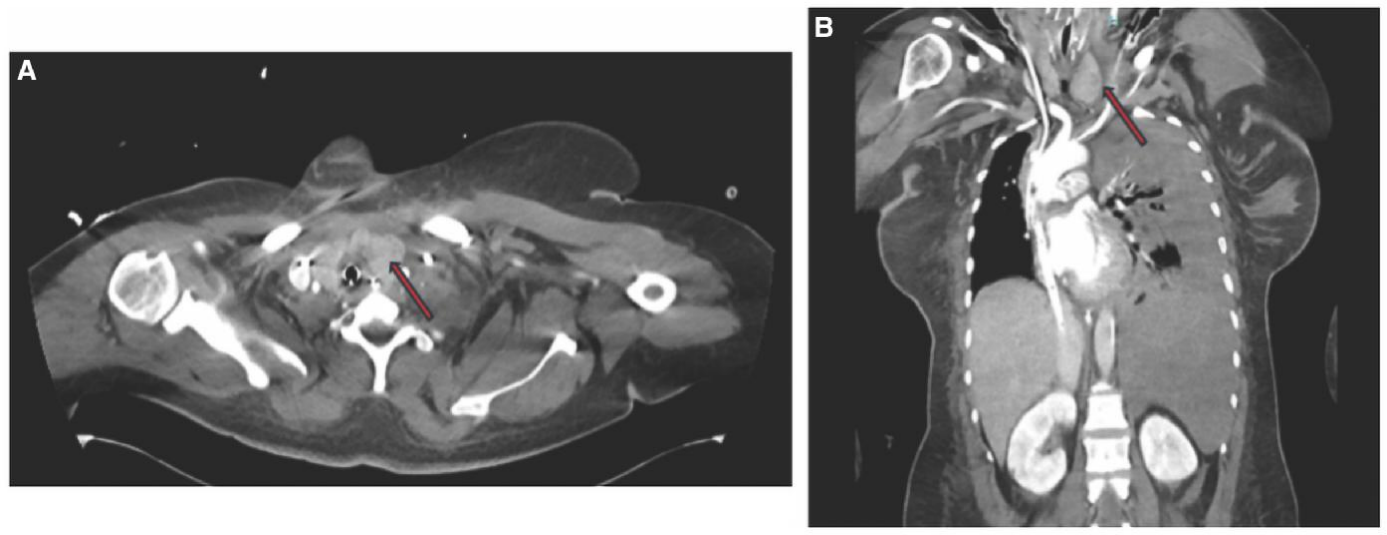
Computed tomography chest A, axial and B, coronal view, demonstrating an asymmetrically enlarged left thyroid lobe.
